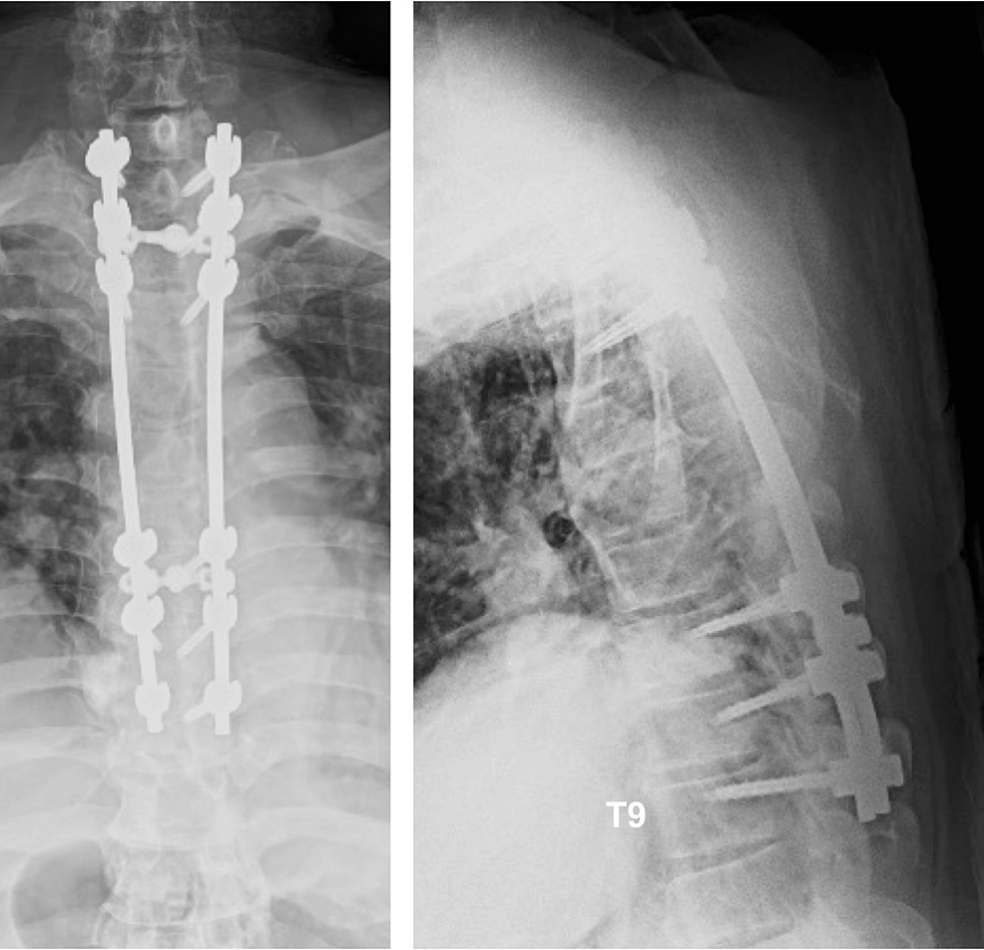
Radiographs after initial surgery Radiographs after posterior decompression and fixation at T1–9, along with laminectomy and OLF resection without instrumented fusion at T10–11. OLF: ossification of the ligamentum flavum
